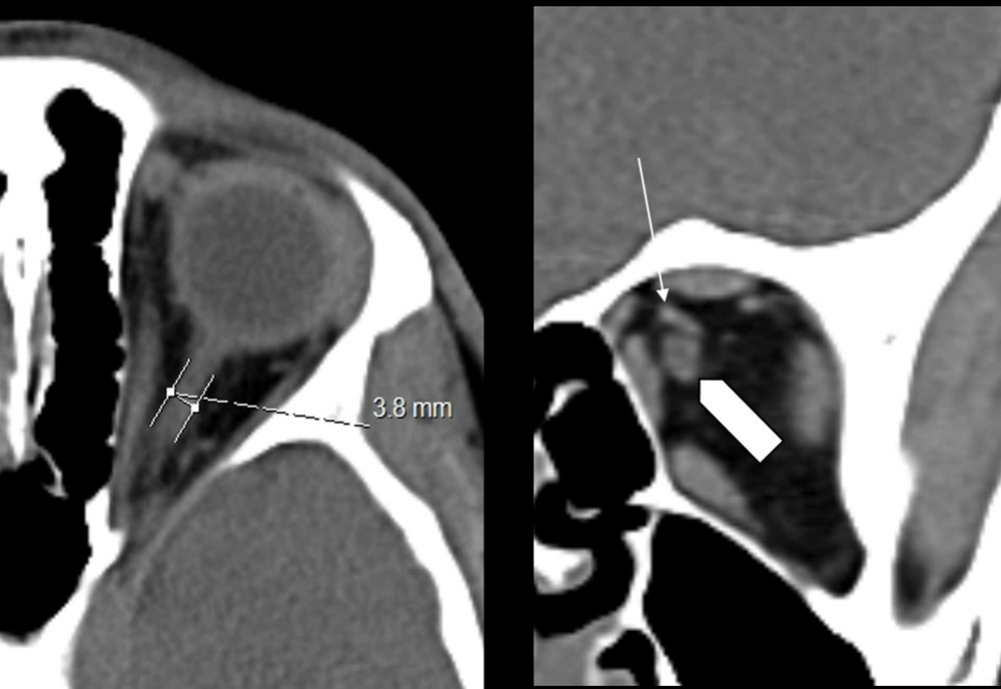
ONSD measurements. A. The measurement of the optic nerve sheath diameter on axial noncontrast CT scan at a point where the ophthalmic vein (thin white arrow) crosses the optic nerve (thick white arrow). B. Coronal images used as reference. The white arrow shows the ophthalmic vein crossing the optic nerve.
